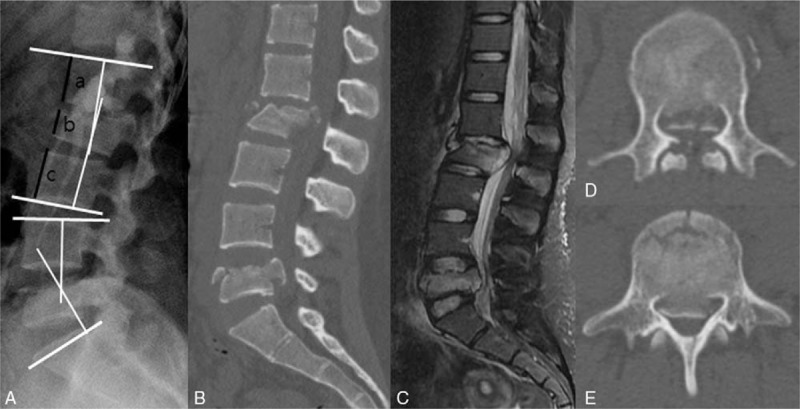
Preoperative radiologic analysis. (A) The Cobb angle of L2 and L5 was formed between white lines were 3.8° and −32.8°, respectively. The vertebral body compression calculated by the formula being [1−(2b/a + c)] × 100. Calculation results were 40% at L2 and 50% at L5. (B) The preoperative sagittal CT scan showed severe obliteration of the spinal canal. (C) Preoperative MRI scan demonstrated traumatic disc injury at L1-2 and acute burst fractures at L2 and L5. Before surgery, canal compromise of L2 (C) and L5 (D) was 70% and 50%, respectively. CT = computed tomography, MRI = magnetic resonance imaging.
